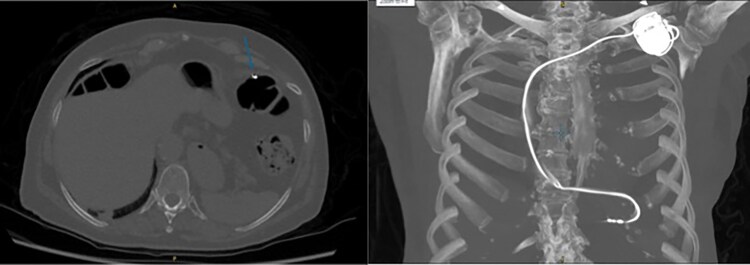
CT scan demonstrating displaced pacemaker lead into the abdominal cavity.
